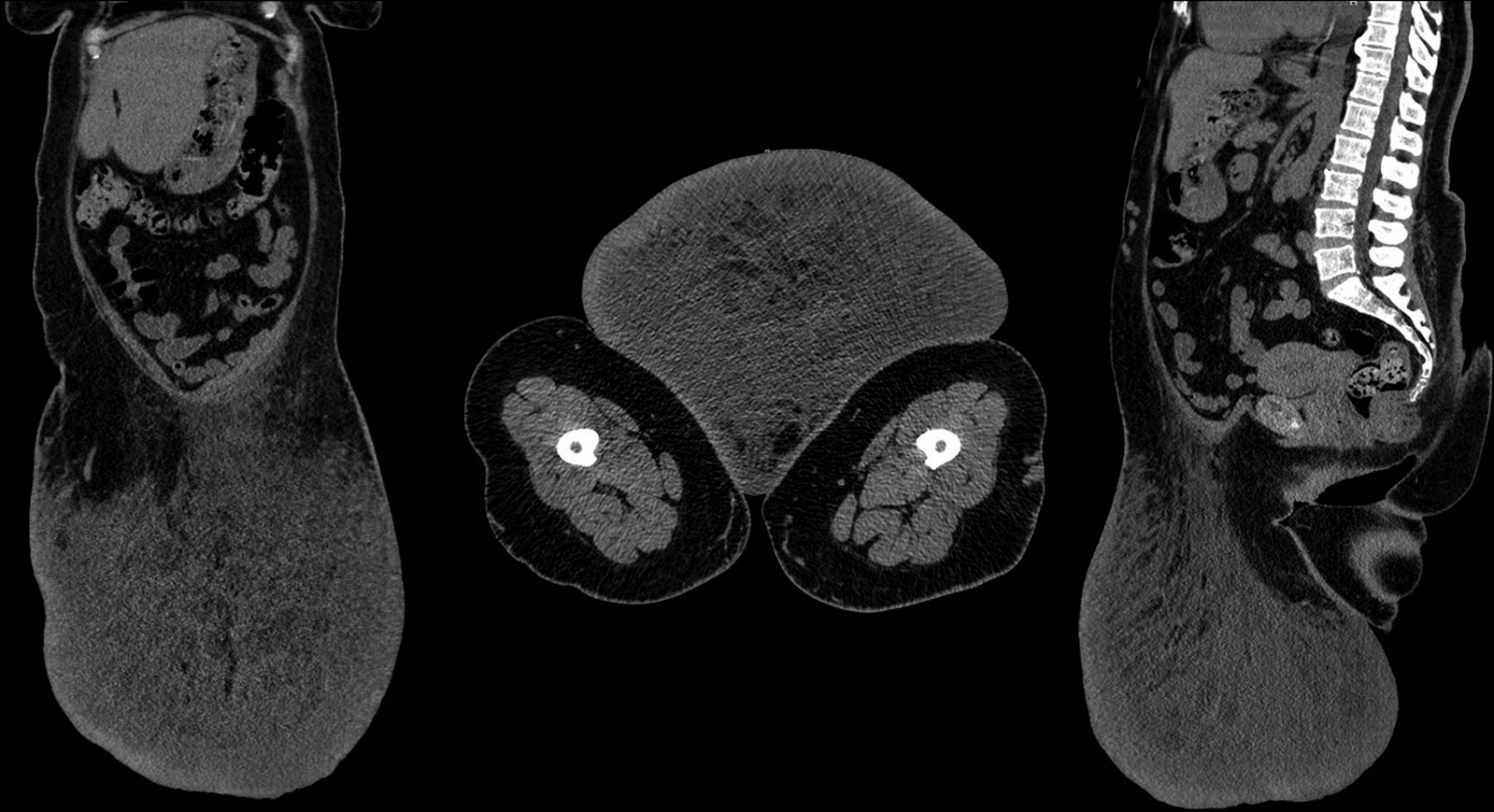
CT-scan of the anterior abdominal wall MLL.
